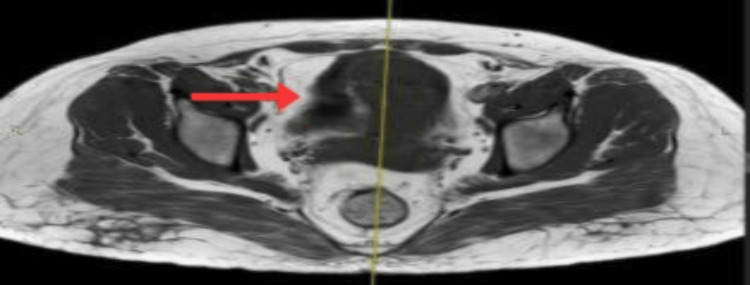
Axial T2-weighted MRI of the pelvis showing a complex left adnexal mass (red arrow) with cystic hyperintense and solid intermediate-signal components. The mass shows both solid and cystic characteristics, with hyperintense fluid content, further indicating a complex ovarian tumor consistent with perivascular epithelioid cell tumors (PEComa).
